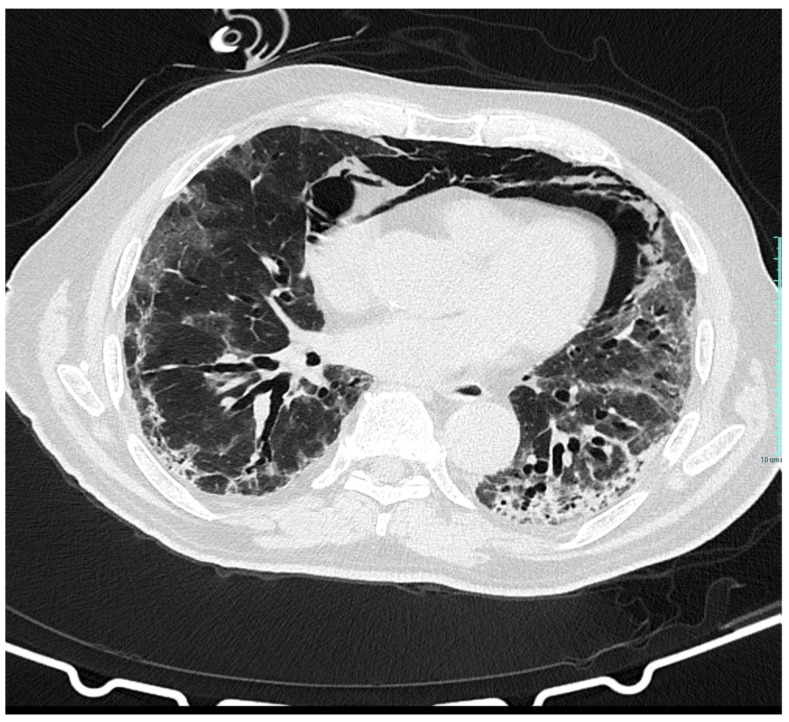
Coronal CT image shows multiple mixed ground-glass opacities in both lungs and pneumomediastinum.
